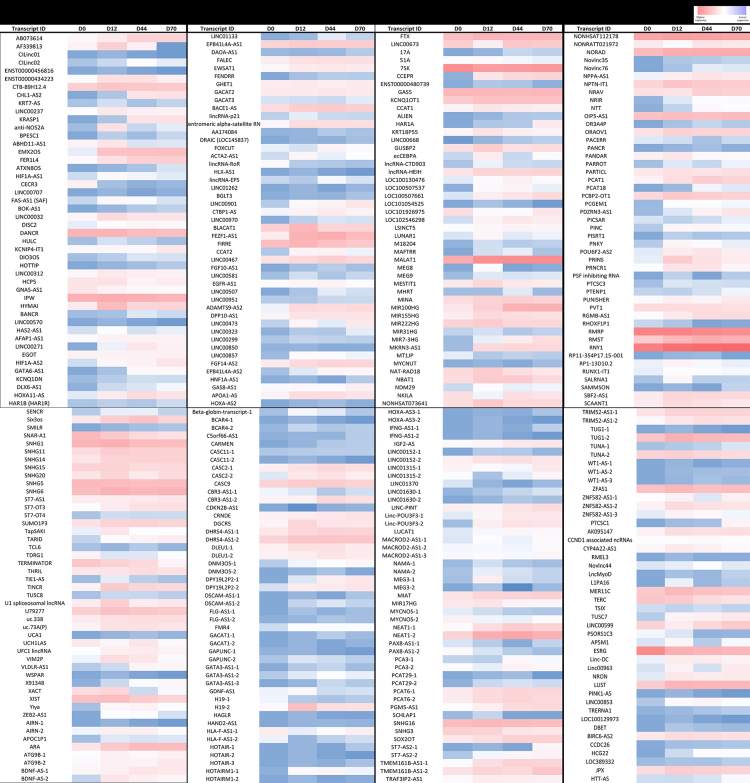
Expression profile of lncRNAs from different stages of *in vitro* embryonic neuorgenesis. The expression of 372 functionally known lncRNAs was analyzed by RT-qPCR using ‘nrStar™ Human Functional LncRNA PCR Array’ on H9 cells at days 0, 12, 44 and 70 of neural differentiation. The delta CT values for individual lncRNAs are shown after normalization to 18S rRNA. The bright red color indicates highest expression whereas bright blue shows lowest expression.
